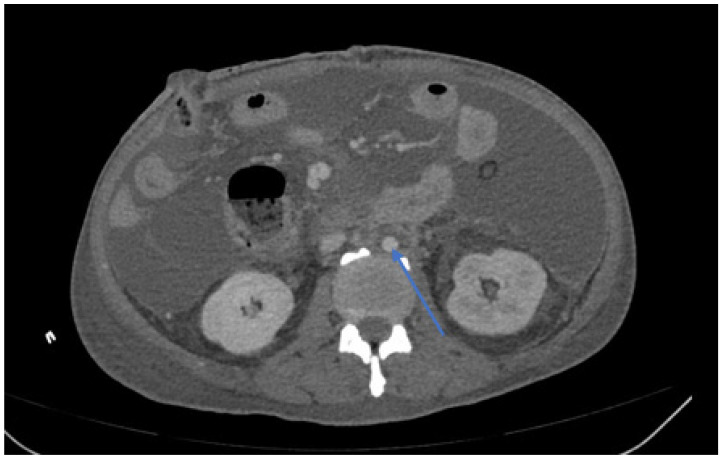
Post-operative CTA image of an abdominal stent graft infection in patients who underwent ISR and subsequent stent graft removal. CTA image at 6 months after ISR surgery indicated that the autologous vein graft was patent (blue arrow).
